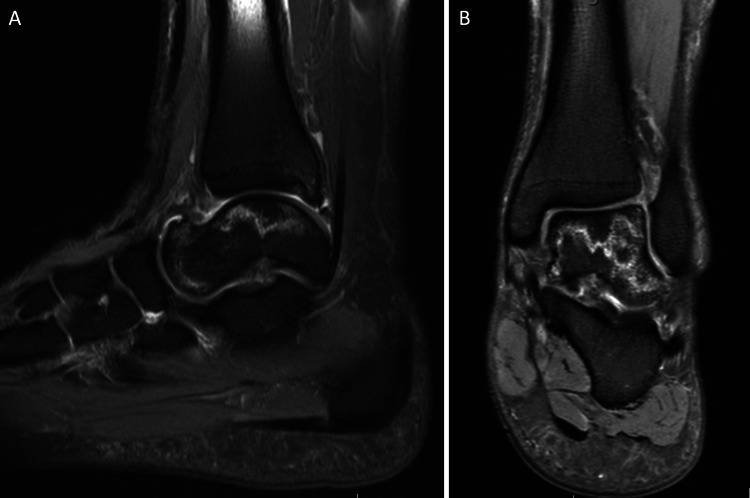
MRI of the left ankle at nine months - June 29, 2021. (A-B) T2-sequence, sagittal, and coronal views show a hyperintensity line with similar signs of osteonecrosis and preserved articular surface. The posterior tibial plafond re-demonstrates a stable small osteochondral defect.
